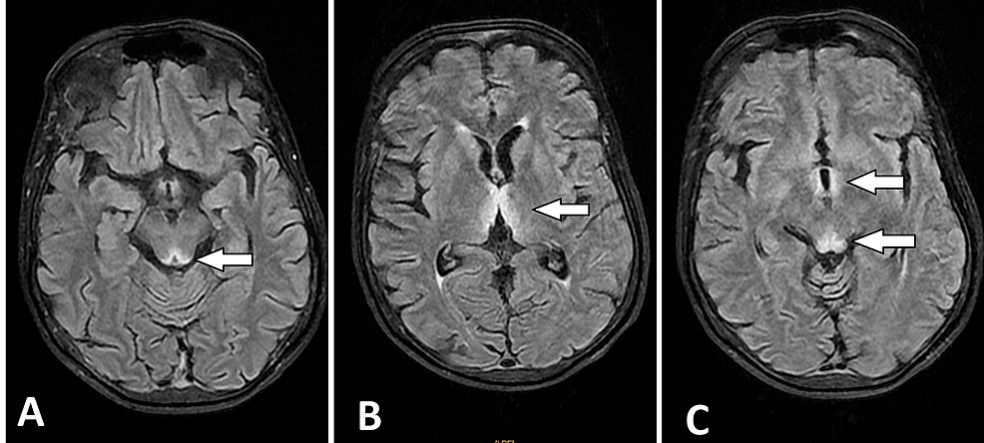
Axial sections of brain MRI In fluid-attenuated inversion recovery (FLAIR) sequence Symmetrical high-signal intensity of the tectum of the midbrain, the periaqueductal gray matter (A), the medial thalami (B) and periventricular region of the third ventricle (C)
